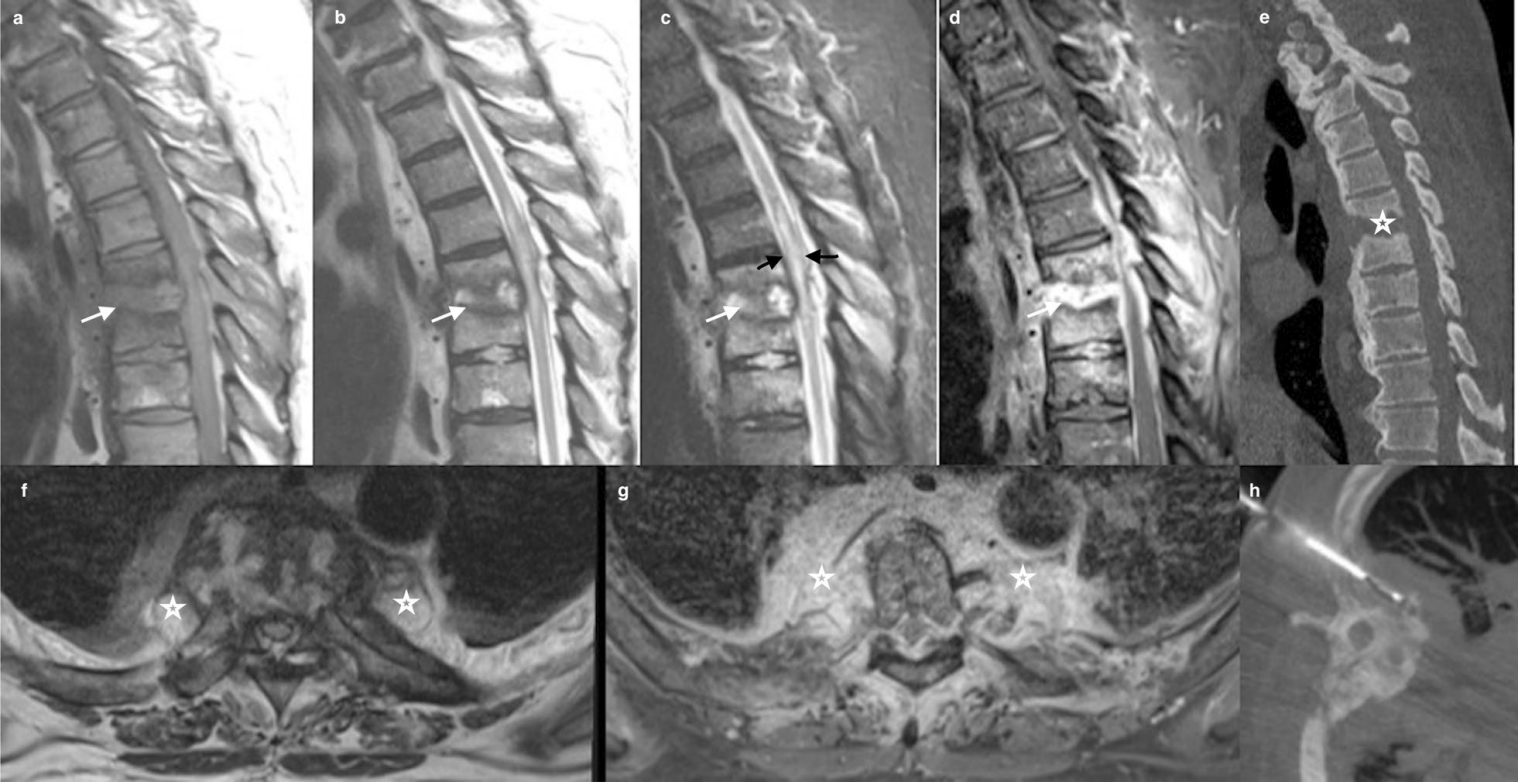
R1 point 9: 78-year-old male with sepsis and thoracic back pain with ankylosing spondylitis. MRI (sagittal T1 (a), T2 (b), STIR (c) and T1 Fat Sat Gadolinium (d)) shows T6-T7 disc hypersignal, enhanced after administration of contrast (arrow) and marked inflammatory surrounding tissue in axial T2 (f) and axial T1FS with contrast (g) (star). Note the elevated medullary signal intensity secondary to compression (black arrow). Reformatted CT (e) shows ankylosed spine and transdiscal fracture (star). Discal biopsy (h) was performed to exclude an infection R1 point 10. Andersson discitis was diagnosed
